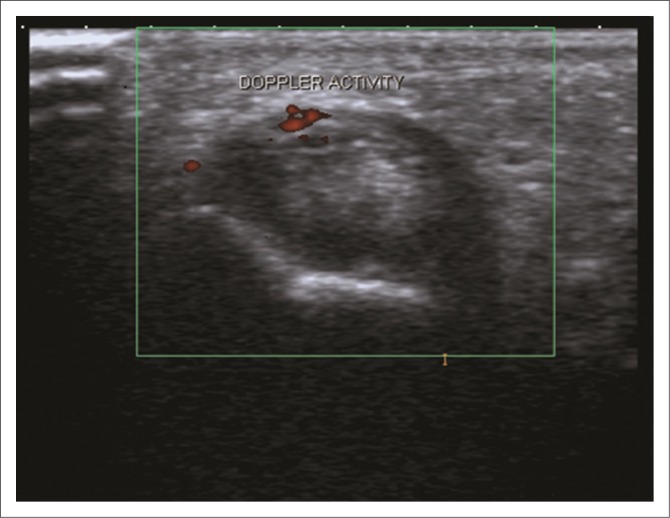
Transverse Doppler ultrasound demonstrating positive Doppler activity in the tendon sheath in keeping with active disease.
